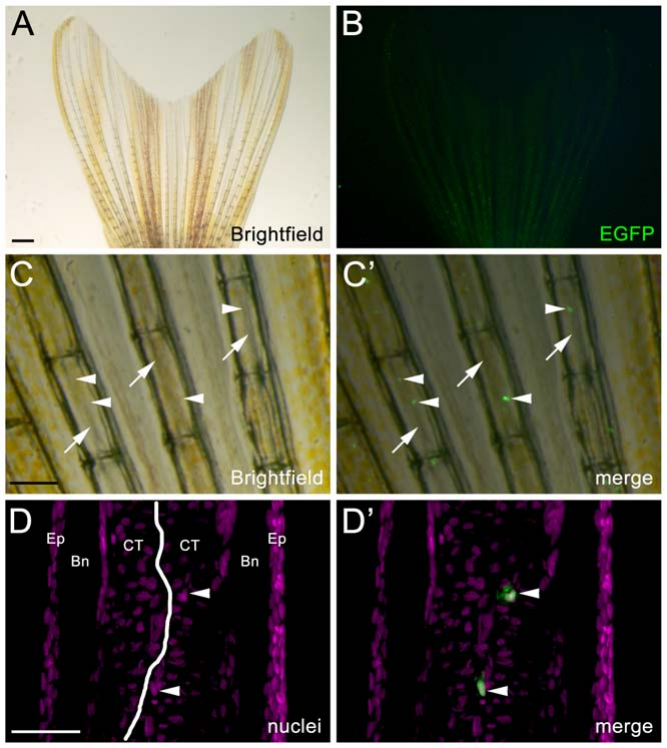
Wholemount brightfield and flourescent images showing *nrd:egfp* transgene expression in the adult caudal tail fin. (A) Brightfield image of the adult caudal fin. (B) Corresponding fluorescent image to panel A. EGFP expression is visualized in the nerve coursing through each bony hemiray of the caudal fin, however at this level of magnification, it is difficult to visualize. (C–C′) High magnification brightfield and corresponding fluorescent overlay showing multiple bony lepidotrichia. The arrows point to the nerve running within each bony hemiray and arrowheads point to EGFP-positive ganglia associated with the nerve. (D and D′) A section of a single bony ray immunolabeled with EGFP to show the transgene and co-labeled with TO-PRO-3 to show all nuclei (magenta). The white line in Panel D shows the location of the nerve. Ep = Epithelium, Bn = bony ray, CT = connective tissue.
